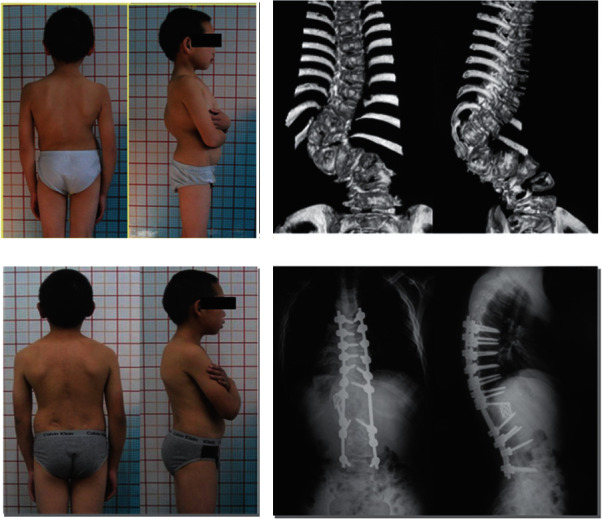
The patient was a 9 years old boy with lumbar congenital scoliosis. (a) Clinical feature preoperative. (b) Computer tomographic with 3-dimensional reconstruction showed multiple complex congenital deformity. (c) Clinical feature at 2 years follow-up after PVCR. (d) X-ray of anterior-posterior and lateral at 2 years follow-up.
